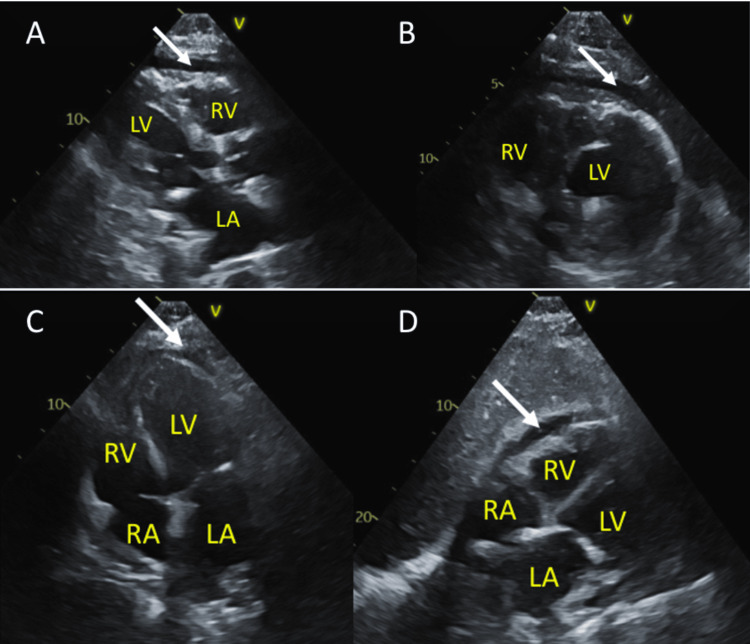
Pericardial effusion on transthoracic echocardiogram. Small pericardial effusion (<1 cm in diameter) is indicated by white arrows. Despite its small volume, the rapid accumulation of fluid resulted in obstructive physiology. Also note the absence of right ventricular collapse. (A) Parasternal long-axis view. (B) Parasternal short-axis view. (C) Apical four-chamber view. (D) Subxiphoid view LA: left atrium; LV: left ventricle; RA: right atrium; RV: right ventricle
